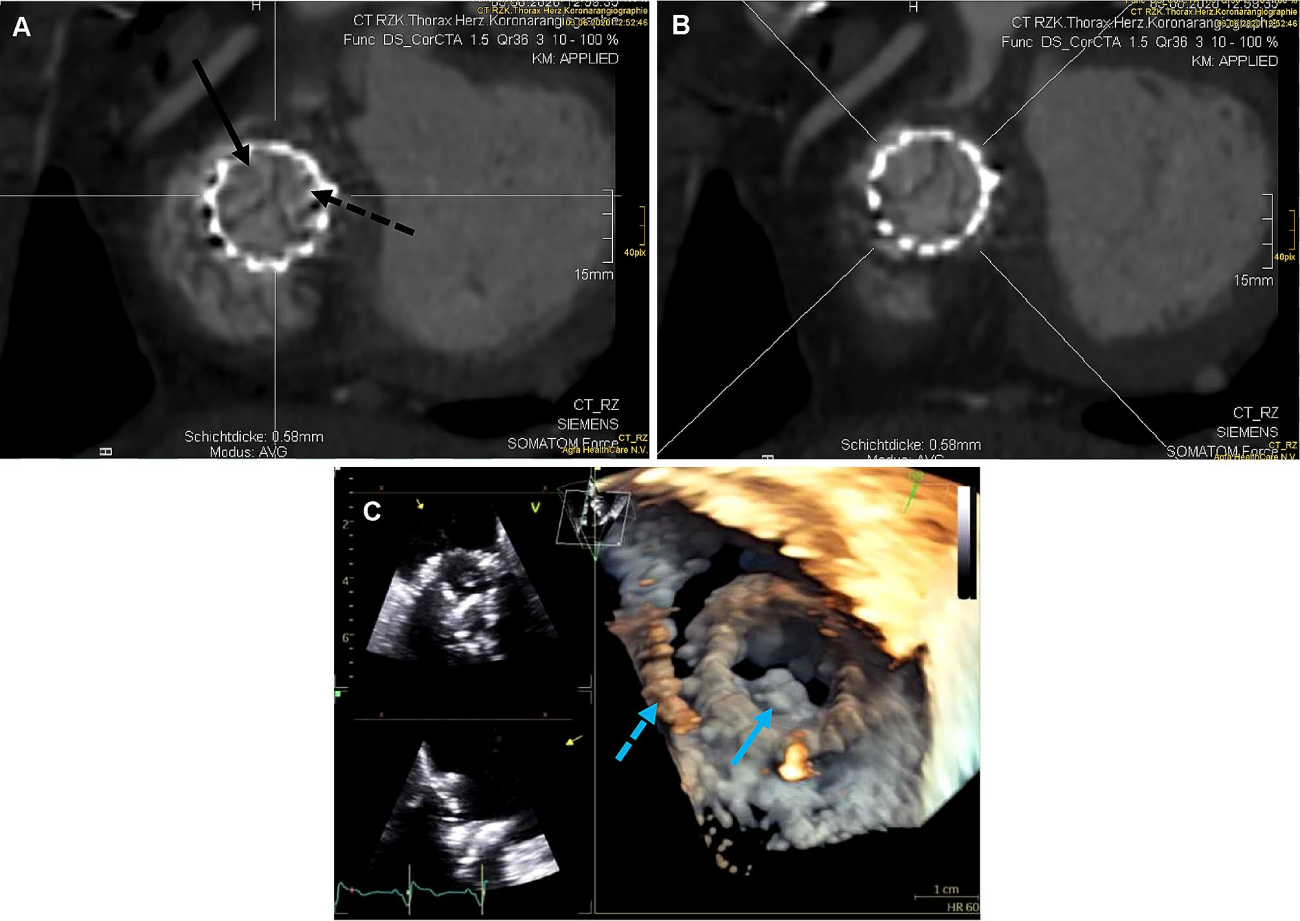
4D-CT and transesophageal echocardiography showing the dysfunctional transcatheter implanted valve-in-valve prosthesis in tricuspid position. a During ventricular systole the leaflets of the bioprosthetic valve close. b During right ventricular filling there is no movement of the right cranial valve leaflet (arrow), an impaired movement of the left cranial valve leaflet (dotted arrow) and a normal opening of the caudal leaflet. c Three-dimensional transesophageal echocardiography (right-atrial view) of the dysfunctional prosthesis with signs of leaflet degeneration (arrow) and the pre-existing pacemaker lead in place (dotted arrow)
